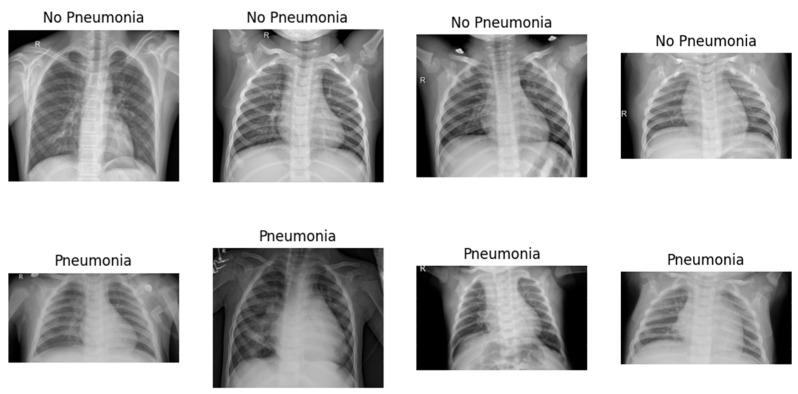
Sample Pneumonia and Non-Pneumonia chest X-ray Images.
